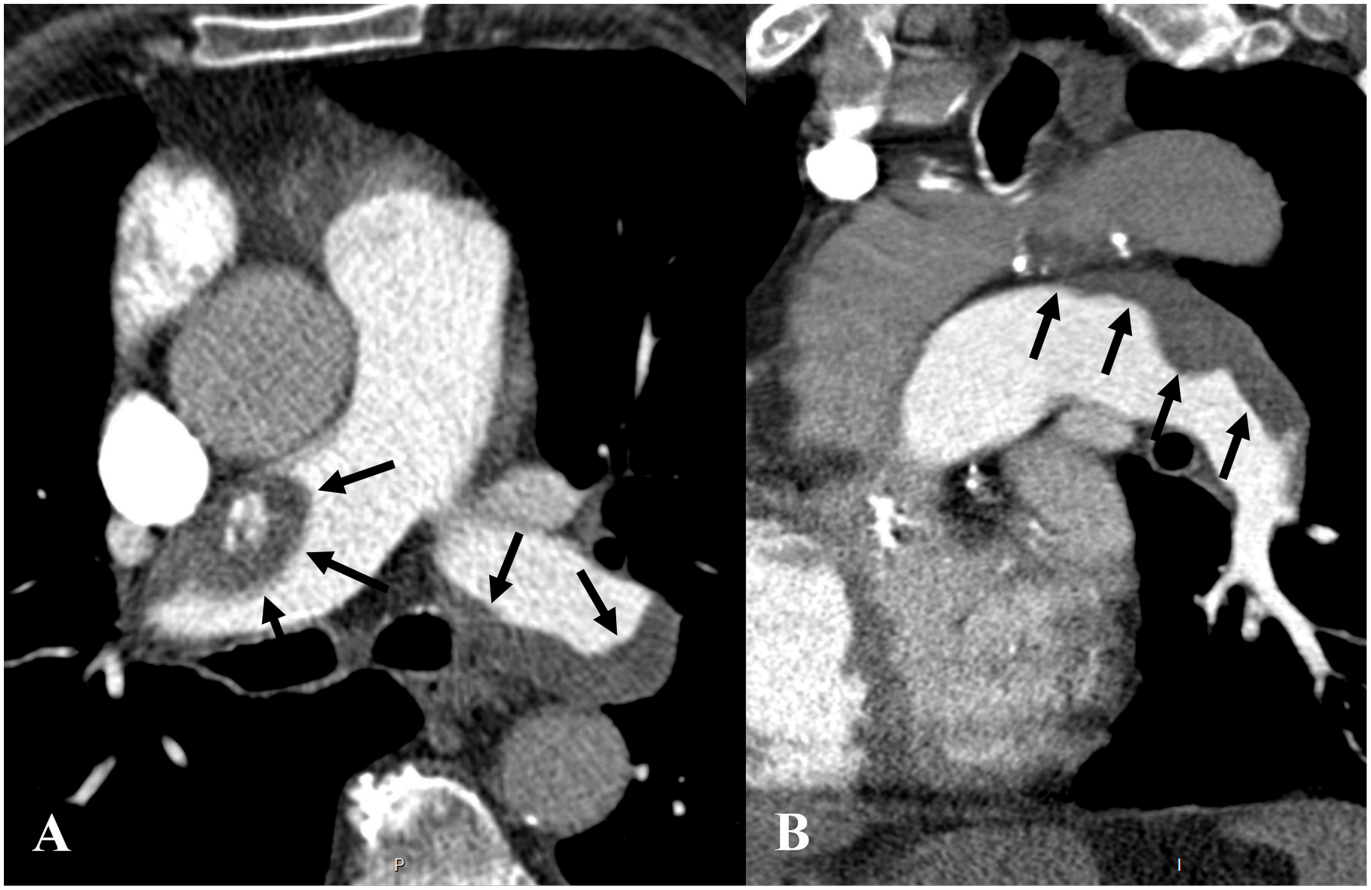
Axial (A) and sagittal oblique (B) CT pulmonary angiography in soft tissue windows in an 85-year-old man presenting with acute and chronic pulmonary embolism. (A,B) show flattened eccentric and mural thrombi (black arrows) with a broad base forming obtuse angles with the vessel wall in both pulmonary arteries, consistent with chronic pulmonary thromboembolism. The radiology technician rated the Qanadli obstruction index (QOI) 50% with high-risk stratification, while the radiologist rated the QOI 17.5% with low-risk stratification.
